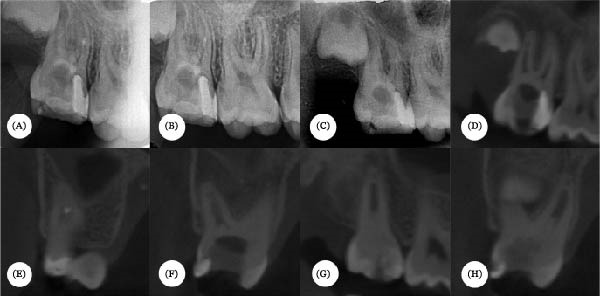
Radiographic and tomographic follow‐up after regenerative endodontic treatment. Follow‐up examinations. (A) Immediate postoperative periapical radiograph. (B) Radiographic follow‐up after 3 months. (C) Radiographic follow‐up after 8 months. (D–H) Cone‐beam computed tomography (CBCT) images obtained after 2 years demonstrating apical closure and evidence of continued root development.
